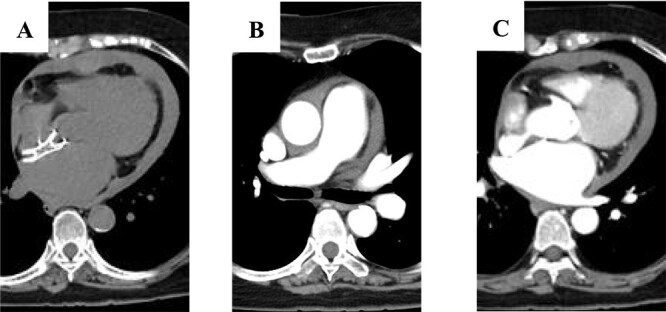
Computed tomography scan showing: (A) circumferential pericardial effusion; (B) no aortic dissection; and (C) no extravascular leakage of contrast media.
